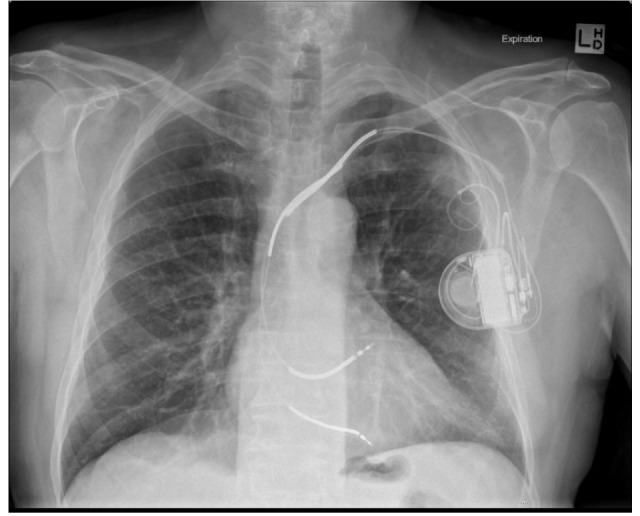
Expiratory chest radiograph taken 2 hours post-procedure shows no pneumothorax despite the patient’s history of COPD.
